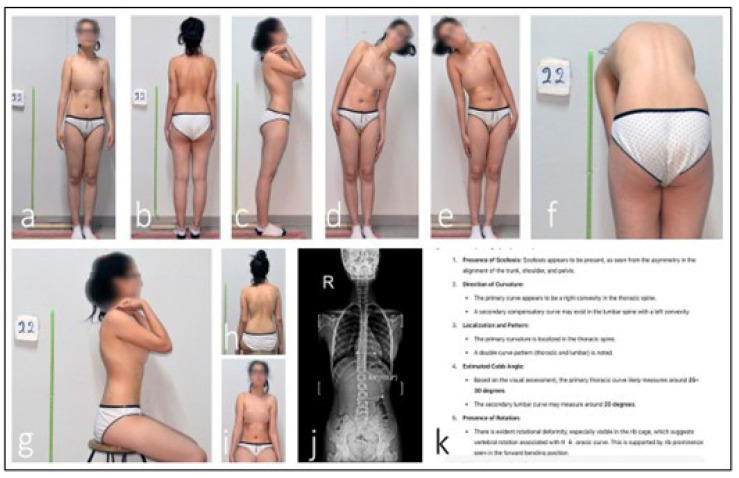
Discrepancy in ChatGPT AI-assisted scoliosis assessment. An analysis of the patient’s clinical photographs by the ChatGPT AI system identified an estimated 25–30° thoracic scoliosis with an interpreted 20° compensatory lumbar curvature (a–h,k). Seated anterior view with neutral shoulder position, used to assess trunk symmetry and anterior rib prominence (i). However, radiographic evaluation measured the lumbar Cobb angle at 6.8°. This discrepancy indicates that the AI system misclassified postural asymmetry as a structural thoracic curve (j).
